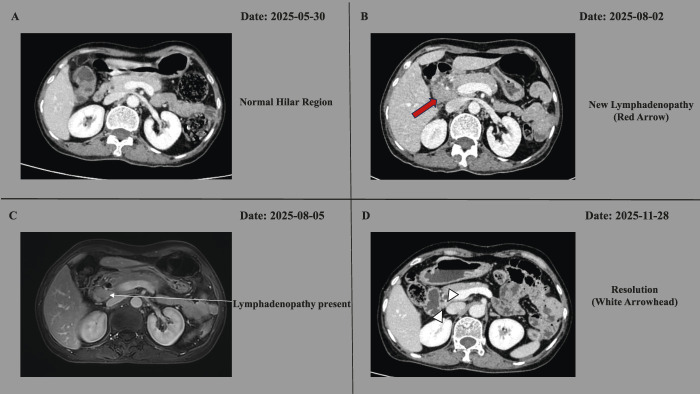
Dynamic radiological evolution of the hepatic hilar region mimicking disease progression. (A) Baseline contrast-enhanced CT demonstrating no enlarged no enlarged portal lymph nodes in the hepatic hilum.(B)CT performed at the onset of jaundice showing a newly developed portal lymphadenopathy, with the largest lymph node measuring 2.7 × 2.3 cm (red arrow). (C) MRI confirming lymphadenopathy, with the largest lymph node measuring 3.1 × 2.0 cm (white arrow). (D) Follow-up CT showing complete resolution of the previously enlarged portal lymph nodes, with no residual enlarged lymphadenopathy identified at the same anatomical location (white arrowhead).
